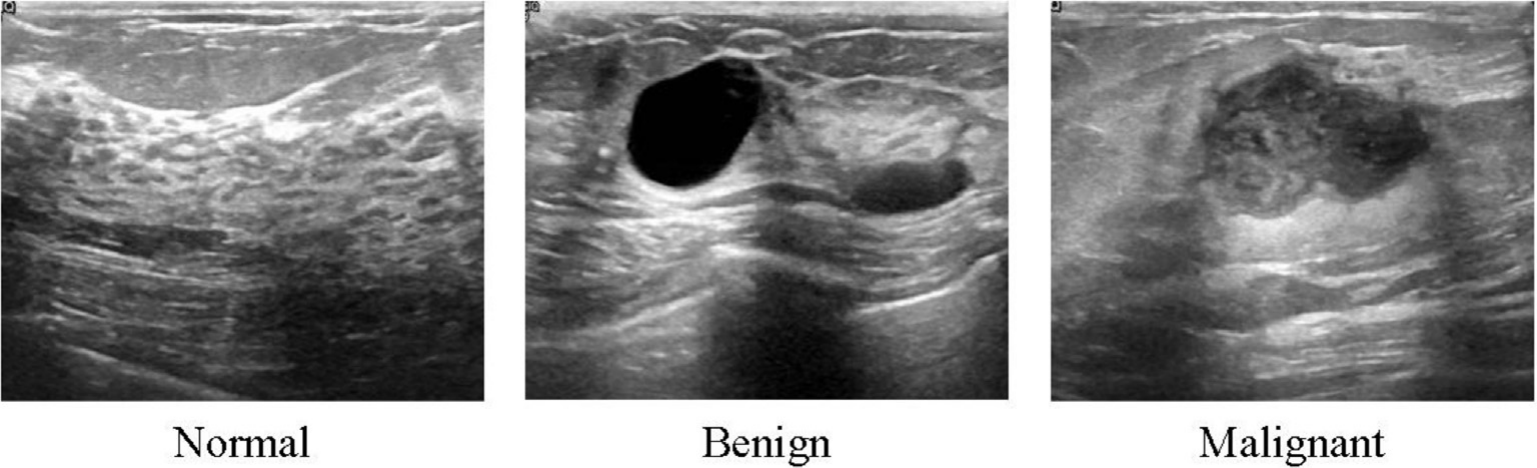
Samples of Ultrasound breast images dataset.
